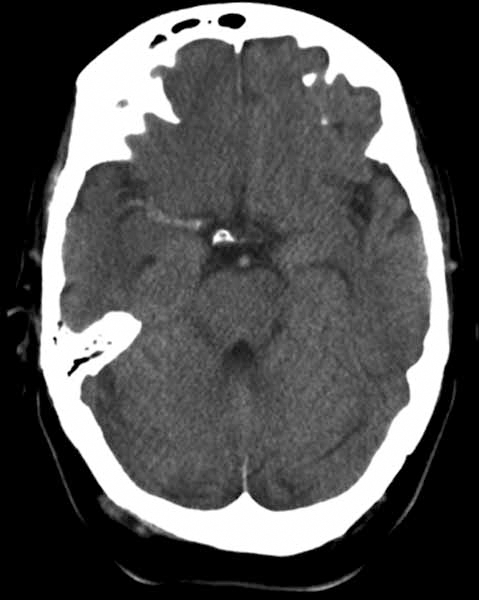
Hyperdense artery sign on CT scan showing a thrombus in the middle cerebral artery.
